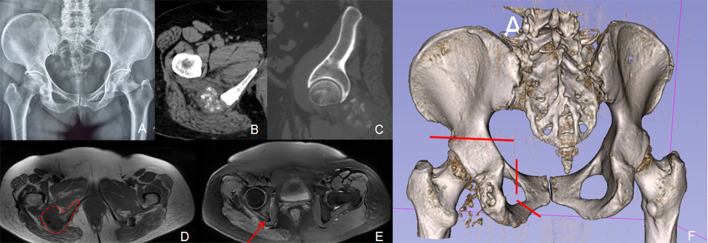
A 54-year-old woman complained of pain in her right buttock. A preoperative puncture biopsy confirmed the diagnosis of chondrosarcoma involving the right ischial tuberosity and the posterior margin of the acetabulum. (A) Pelvic radiographs displayed abnormal bone in the right ischial tuberosity and the lower margin of the acetabulum, with patchy bone sclerosis and low-density areas visible within. (B, C) CT scans indicated popcorn-like bone abnormalities in the right ischial tuberosity, involving a portion of the lower margin of the acetabulum. (D, E) MRI scans showing a mass-like lesion with mixed low T1 and high-low T2 signals in the right ischial tuberosity (encircled by the red dotted line), while the posterior margin of the right acetabulum was involved (indicated by a red arrow), with patchy edema reaction bands visible in the surrounding muscles and soft tissues. (F) Preoperative plan: Intended to perform *en-bloc* resection of the ischial and acetabular regions (the red line represents the proposed intraoperative osteotomy line).
